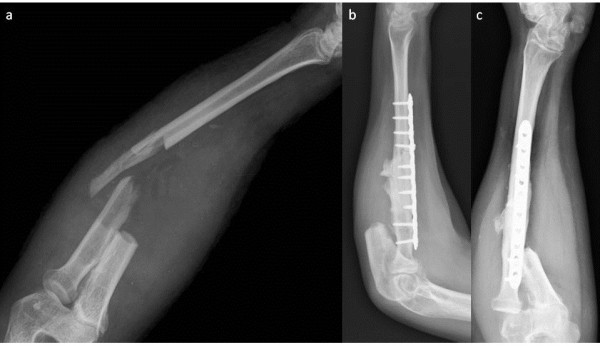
X-ray image of radius fracture (a) and the radius osteosynthesis with plate, screws and PMMA (b, c).
